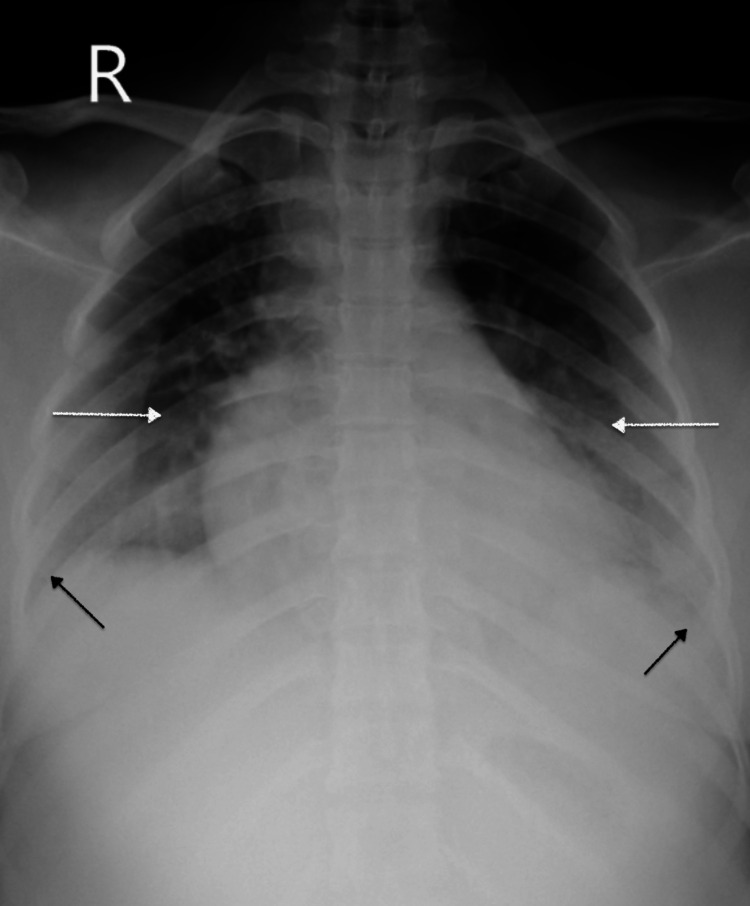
Chest X-ray showing bilateral consolidation (white arrows) with blunting of costophrenic angles (black arrows) suggestive of effusion.
